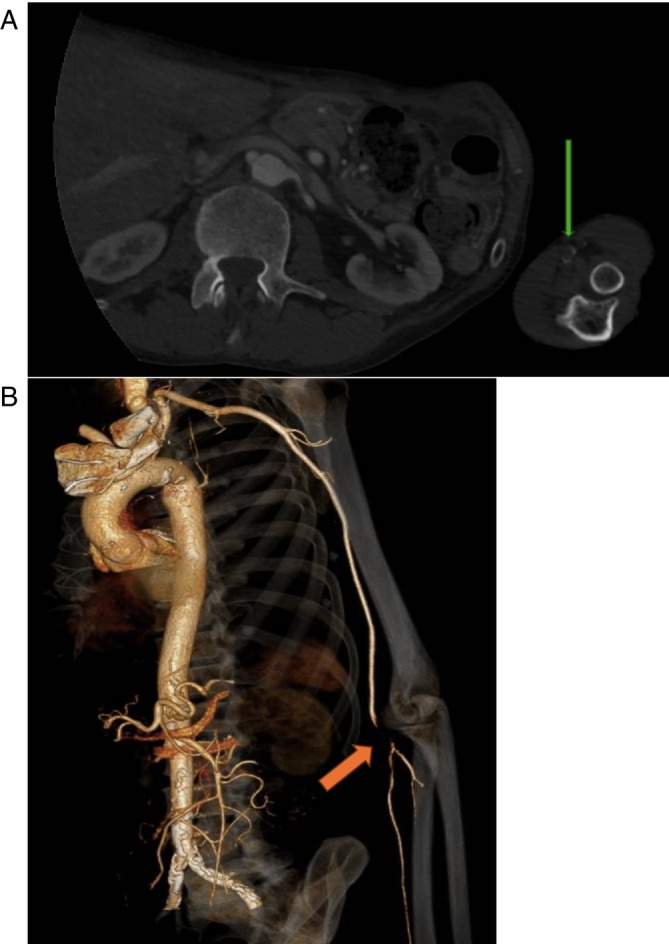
(A) CT Angiography (Arterial Phase) Showing Arterial Occlusion (Axial Slice). The green arrow shows a lack of contrast in distal brachial artery highlighting an occlusion in the artery. This is more easily appreciated in (B). This is a 3D Reconstruction of CT‐A of Upper Limb prior to the patient's first embolectomy. Please note the orange arrow highlighting the absence of continuity from the distal brachial artery to its bifurcation as it becomes the radial and ulnar arteries. This lack of continuity shows the absence of blood flow in the affected area.
